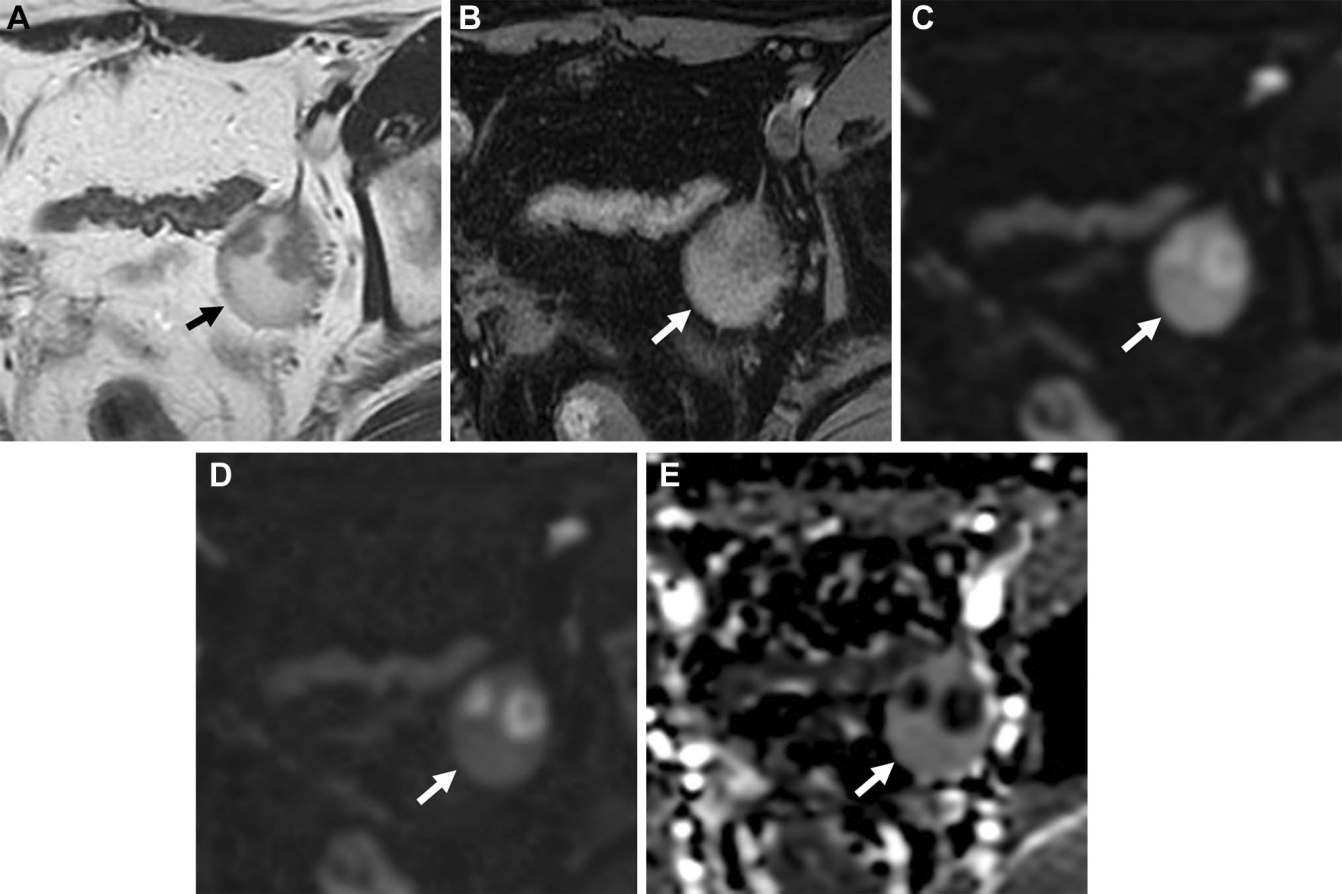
Endometriosis-associated ovarian carcinoma: endometrioid carcinoma. A 35-year-old with suspicion of an ovarian cystic mass with mural nodules on ultrasonography examination. A T2-weighted image, B fat-saturated T1-weighted image, C DWI (*b* = 800 s/mm^2^), D computed DWI with high *b* value (*b* = 1500 s/mm^2^), and E ADC map show a left ovarian cystic mass (arrow) with mural nodules. The cyst contents show high signal intensity on both T1- and T2-weighted images reflecting hemorrhagic fluid. The mural nodules show water diffusion restriction on DWI, however, high signal intensity hemorrhagic cyst contents mask the signal of mural nodules. High b-value computed DWI can reduce the signal of cyst contents and high signal intensity of mural nodules is clarified
